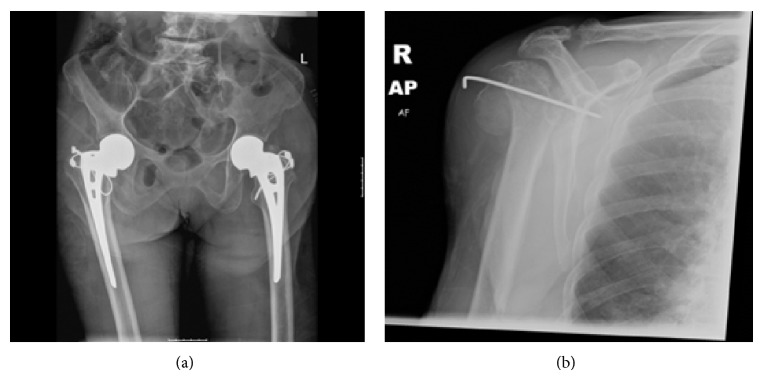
Postoperative radiographs demonstrating (a) bilateral hemiarthoplasties and (b) K-wire stabilisation of the right shoulder.
